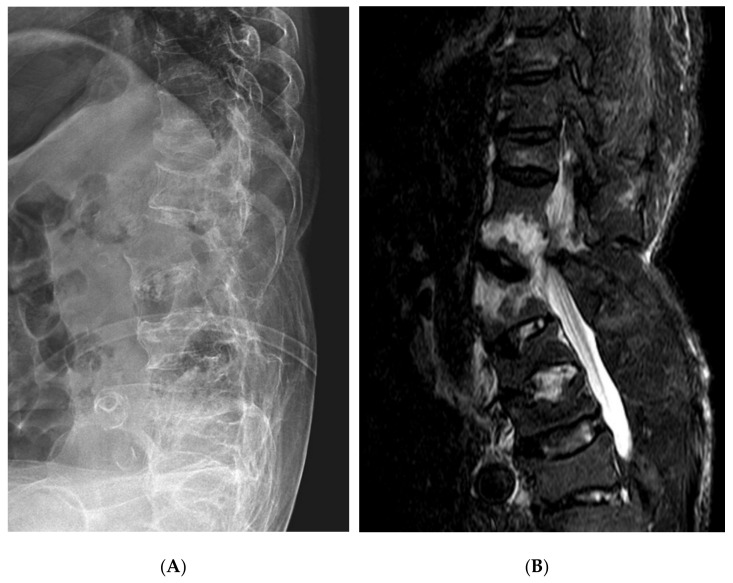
The lumbar spine lateral plain radiograph (A) and MRI sagittal view (B) after fracture.
